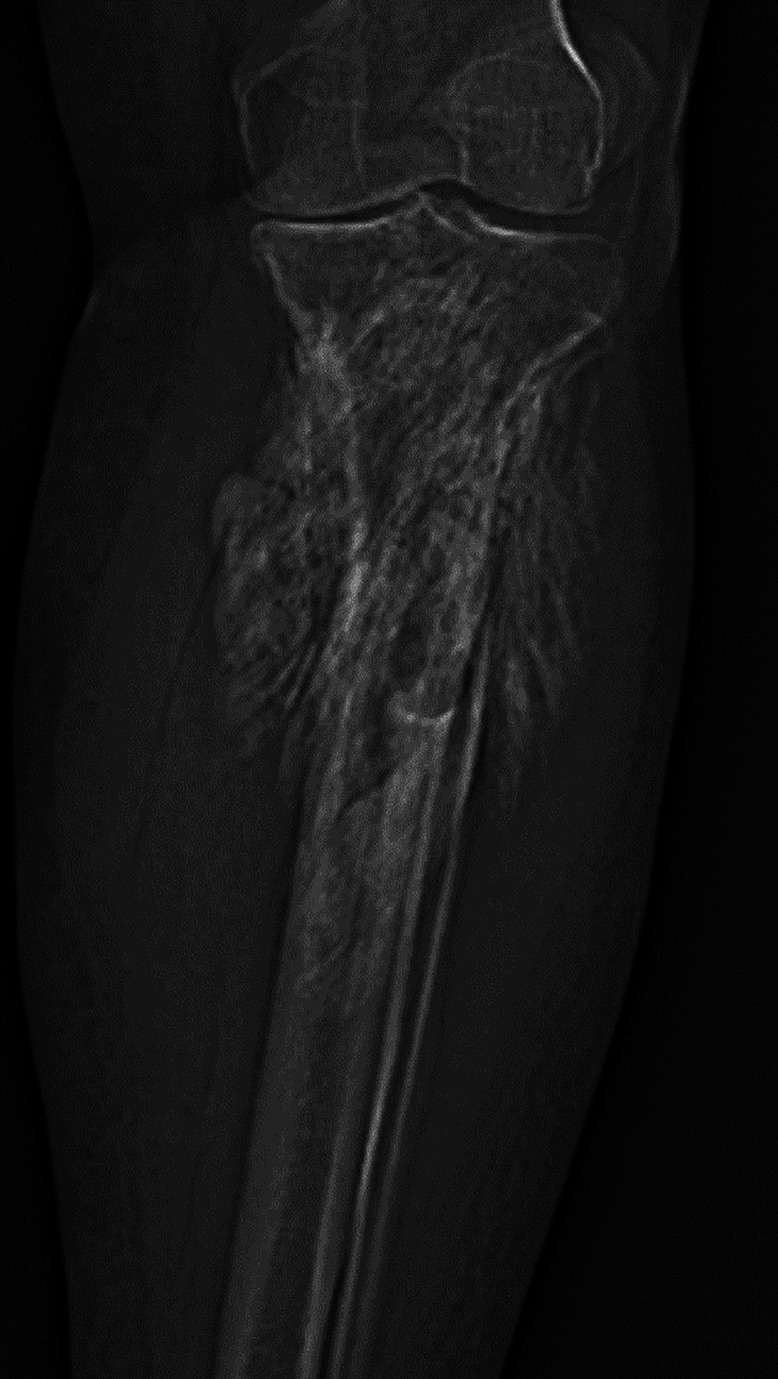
Initial radiograph of the left calf (frontal view) showing dense, inhomogenous, lobulated mass in the proximal tibial region.
